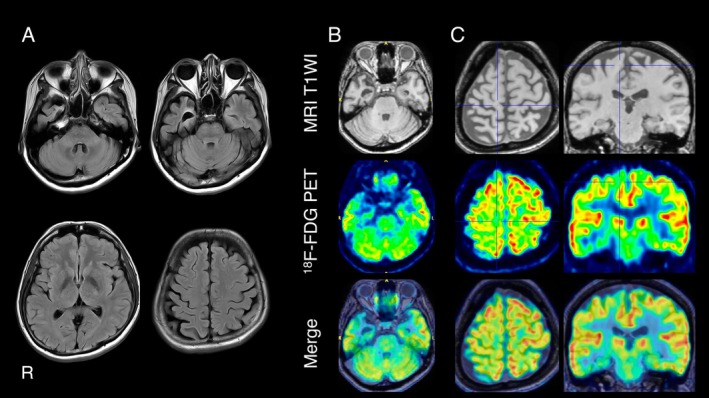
Magnetic resonance imaging and [^18^F]‐2‐fluoro‐2‐deoxy‐D‐glucose positron emission tomography (^18^F‐FDG PET) imaging. (A) Axial fluid attenuated inversion recovery (FLAIR) sequence magnetic resonance images obtained when the patient was at age 57. Atrophy is observed in the anterior and medial regions of the right temporal lobe. (B) ^18^F‐FDG PET brain images show hypometabolism predominantly in the anterior and medial regions of the right temporal lobe. (C) The medial region of the precentral gyrus (indicated by the blue cross mark) shows right‐predominant hypometabolism. The laterality and medial predominance of the metabolic reduction in the precentral gyrus were consistent with the patient's clinical manifestations.
